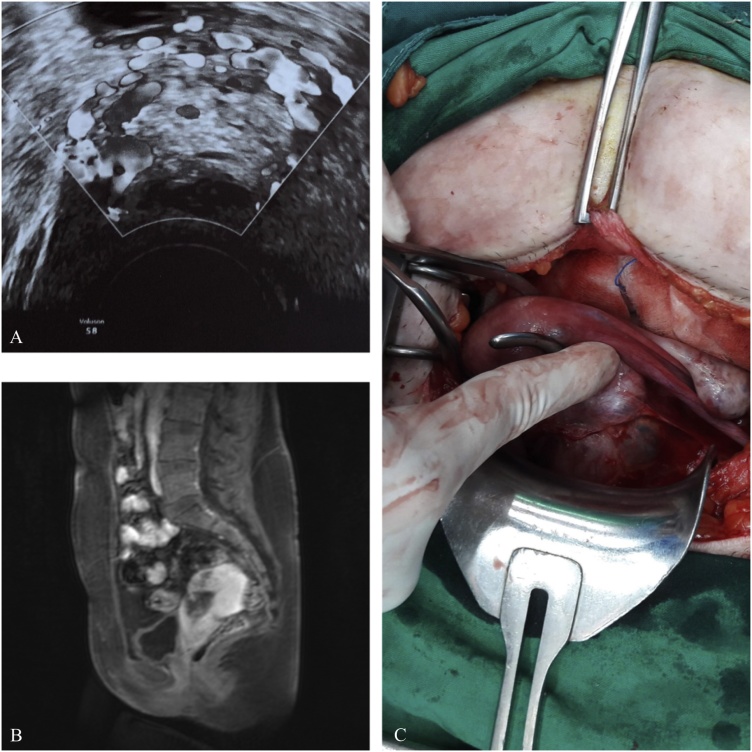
Cesarean scar pregnancy. A. Transvaginal ultrasound imaging of the cesarean scar pregnancy. B. Magnetic resonance imaging of the cesarean scar pregnancy. C. Intraoperative view of the cesarean scar pregnancy.
